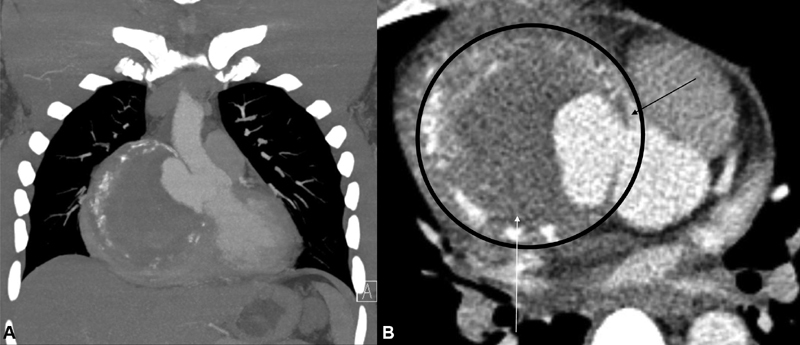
Computed tomography angiography of the chest demonstrating a 10 cm aneurysm of the non-coronary sinus with laminated calcifications. (
